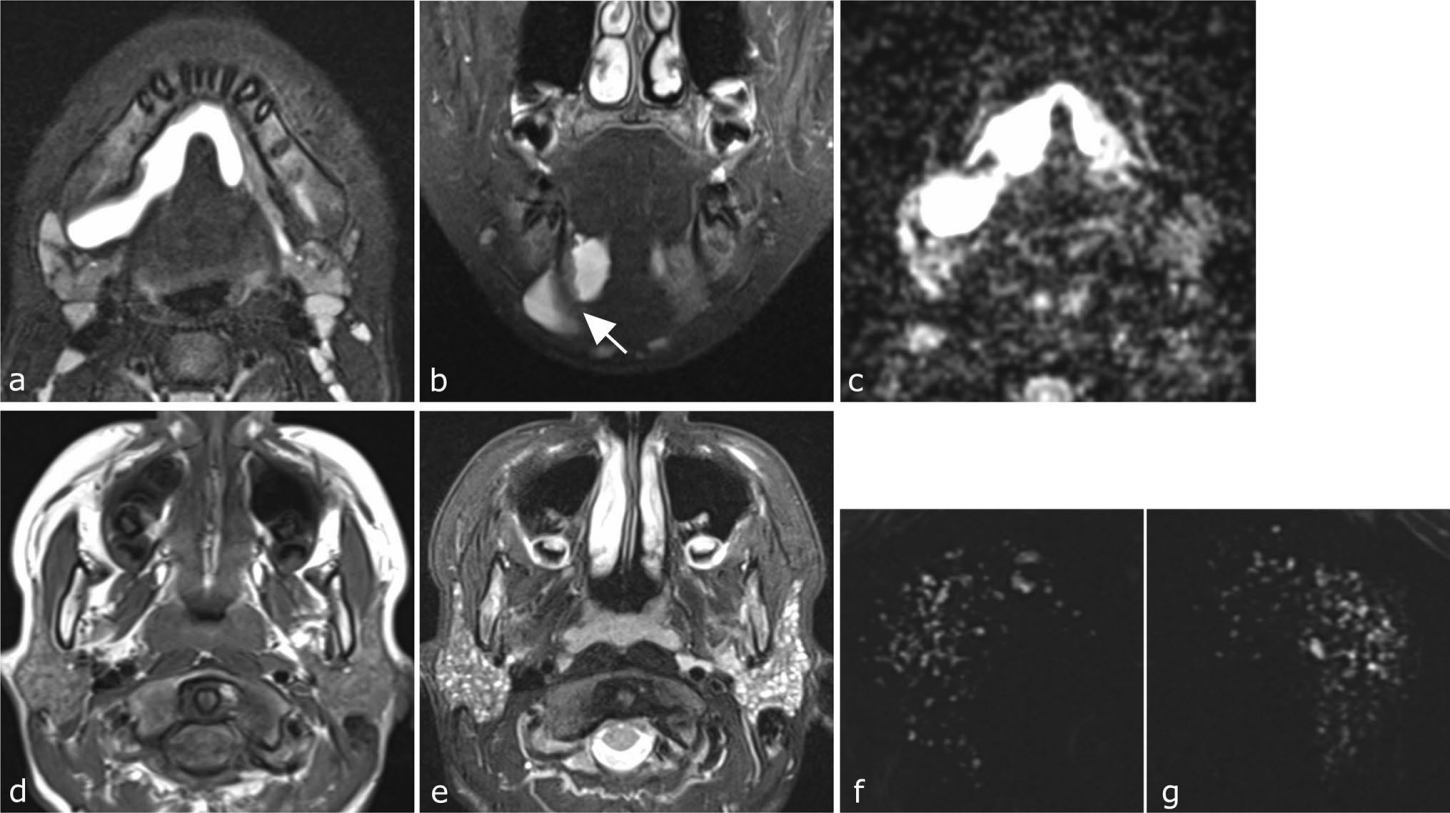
Magnetic resonance images of a 12 year-old boy with plunging ranula who was diagnosed with juvenile pSS (Case 1 in Table 1). a, b Axial (a) and coronal (b) fat-suppressed T2-weighted MR images show a homogeneous high-intensity, irregularly shaped, well-circumscribed cystic lesion extending from the bilateral sublingual space to the right submandibular space beyond the mylohyoid muscle (arrow). c Axial apparent diffusion coefficient (ADC) map shows a cystic lesion with extremely high ADC level. d Axial T1-weighted image shows slightly inhomogeneous parenchyma of the bilateral parotid glands. e Axial fat-suppressed T2-weighted image shows multiple dispersed high-intensity spots in parenchyma of bilateral parotid glands. f, g MR sialography shows punctate sialoectasia in the right (f) and left (g) parotid glands
